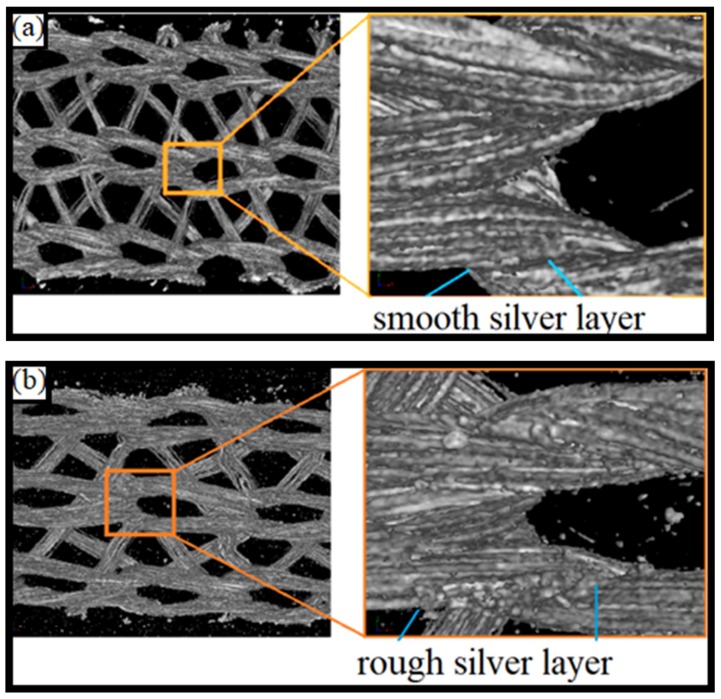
Micro CT as a 3D view of a sample with conductors of knitted textiles: (a) untested reference sample; (b) tested sample after 300,000 cycles at a strain amplitude of 25%.
